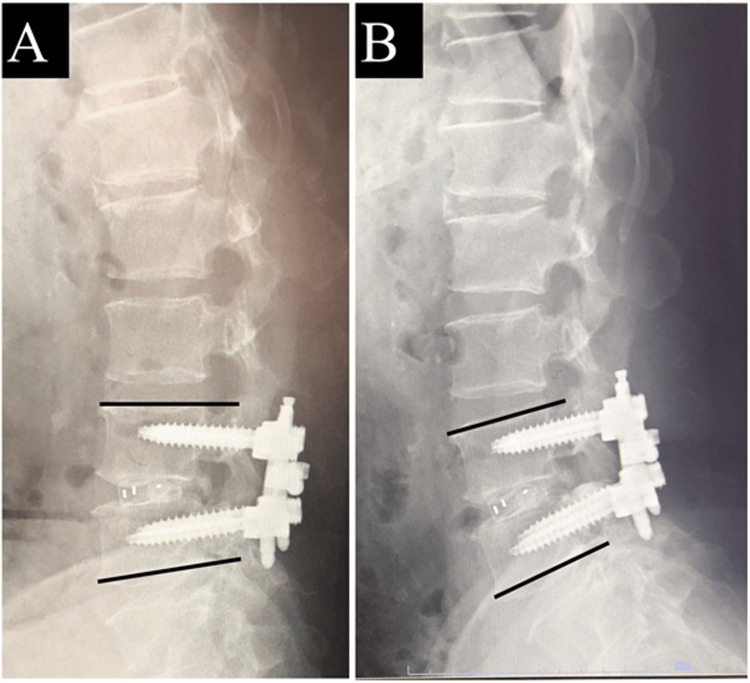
Cobb angle of the vertebral body endplate on the postoperative lateral radiographic image: (A) flexion, (B) extension.
